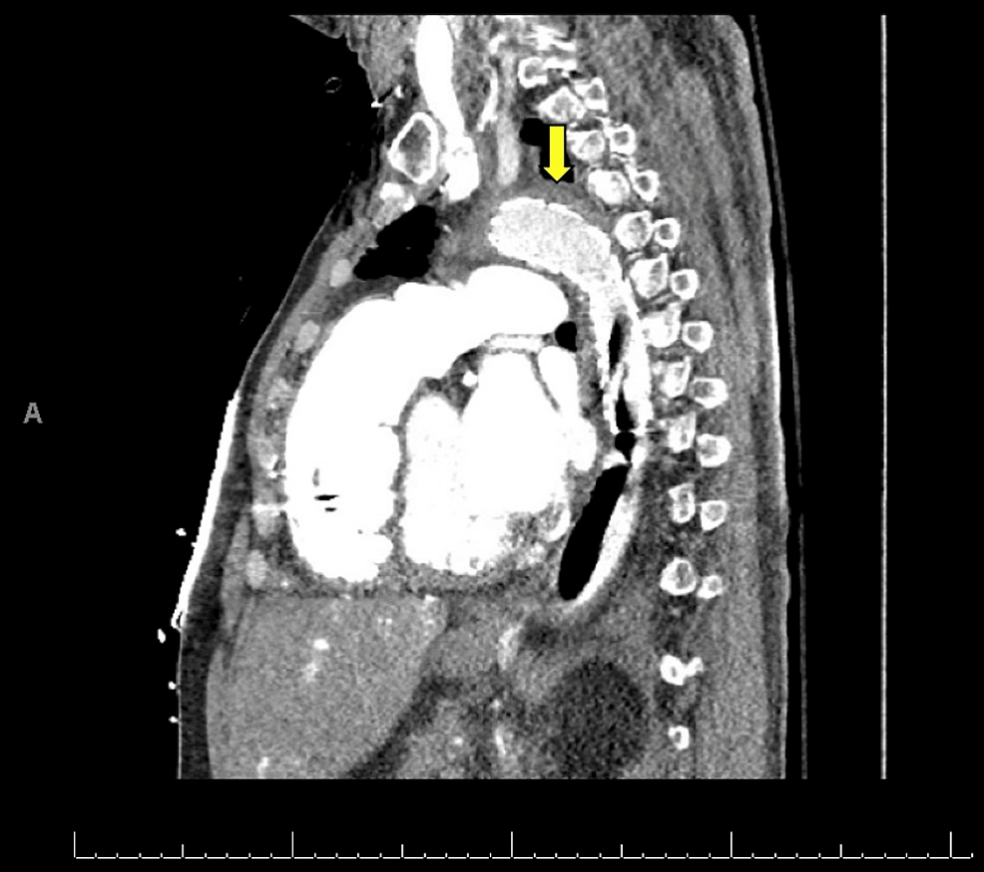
CT angiogram (parasagittal view) demonstrating the presence of a stent graft covering the lesion area at the descending aorta (yellow arrow) and extending distally to cover the intra-aortic balloon pump device.
